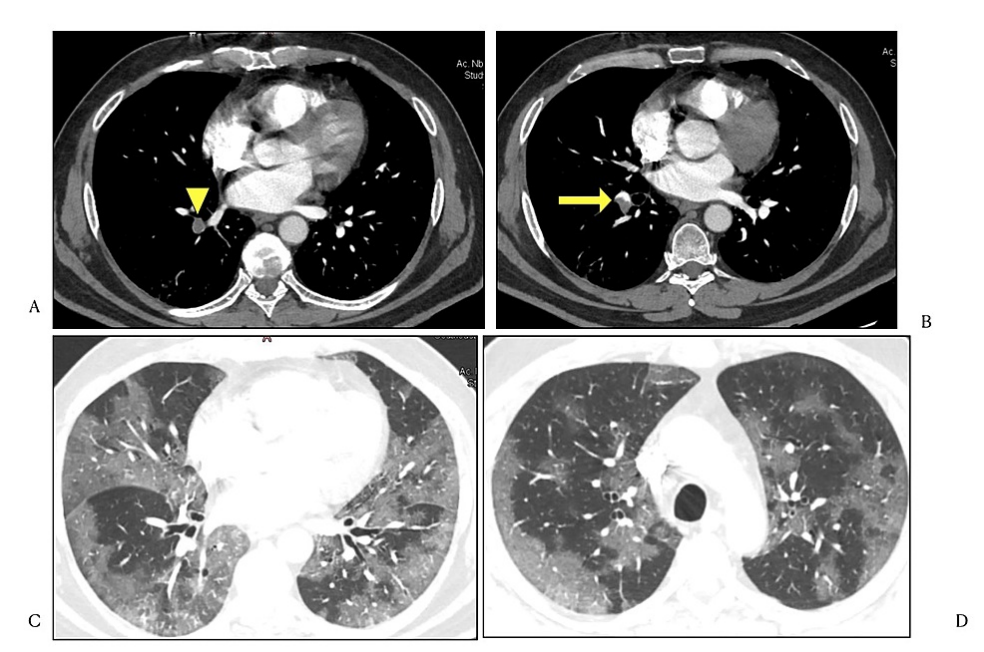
Contrast-enhanced CT Chest (mediastinal window and lung window) axial sections A) and B) show a hypodense filling defect in segmental pulmonary artery of right lower lobe (arrowhead and arrow), axial sections (lung window) show patchy multifocal ground glass opacities with both central and peripheral distribution
